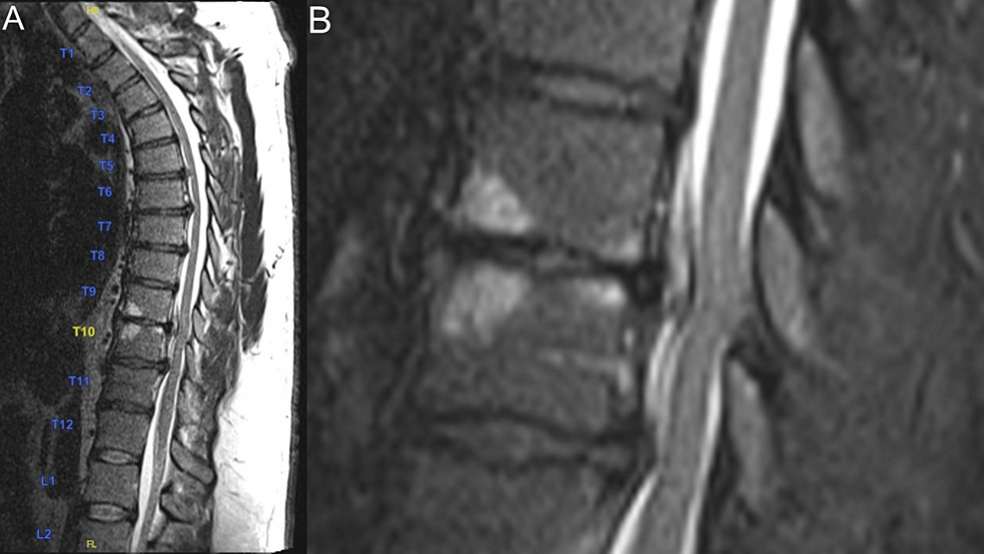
T2 sagittal thoracic spine MRI. (A) T2-weighted midsagittal spine MRI. (B) T2 sagittal inset T9/10/11 1.5x zoom.
